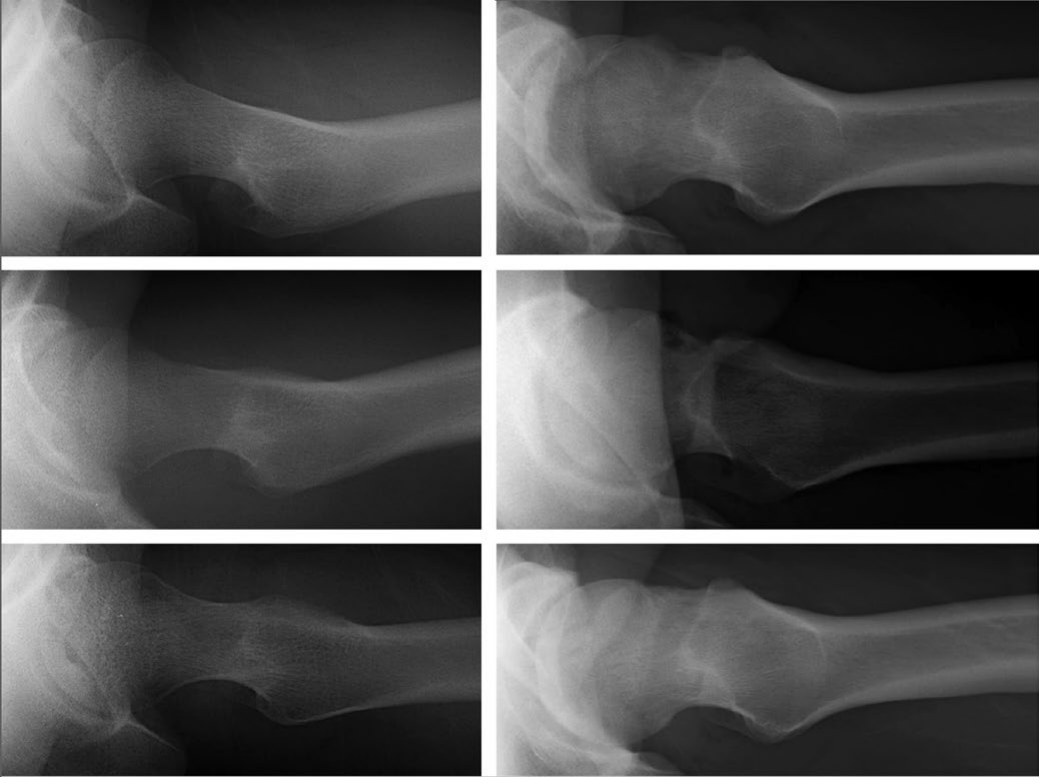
Representative radiographs of cam regrowth.
